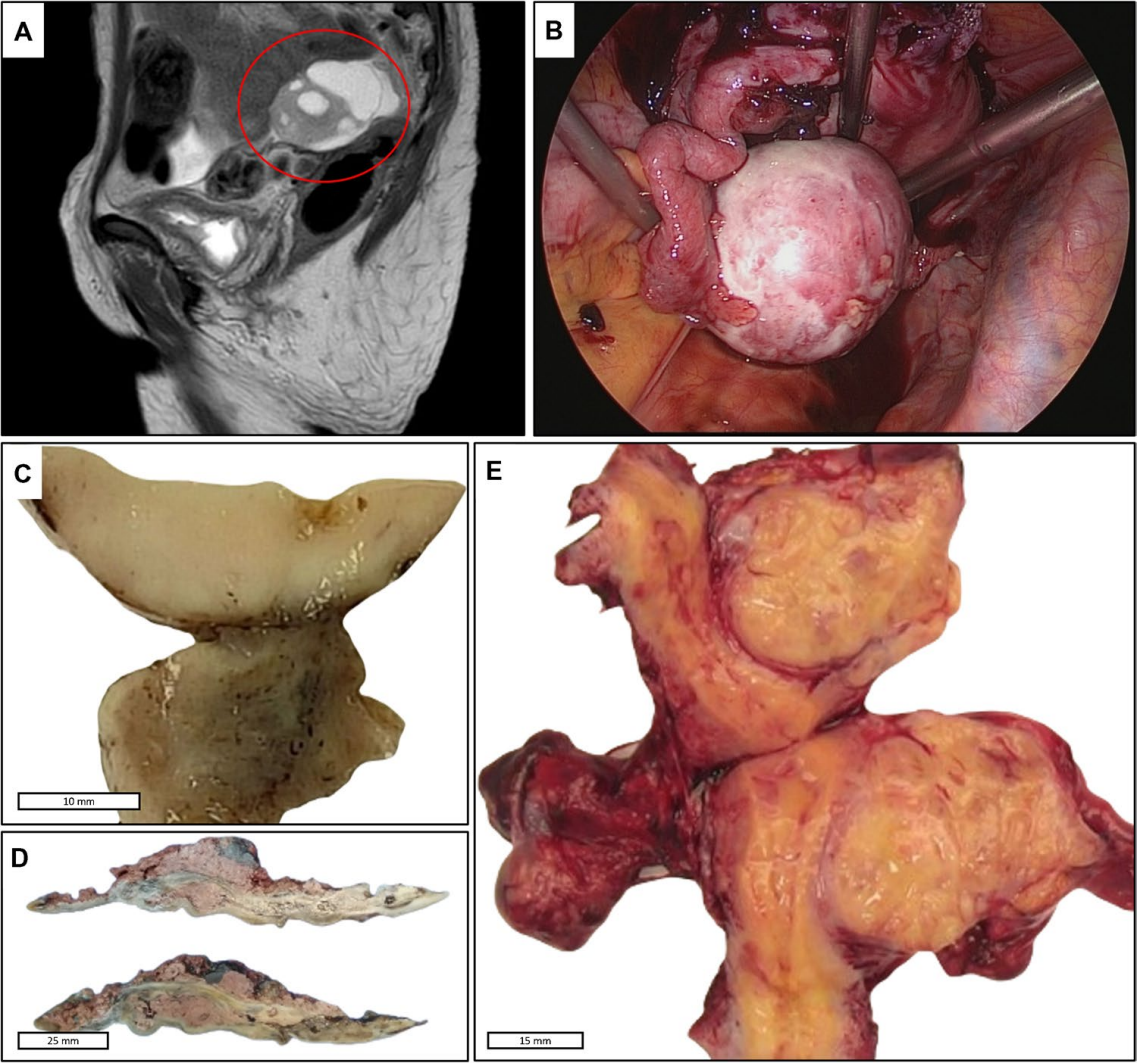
Radiological and gross findings in EWSR1/FUS-CREB-rearranged adnexal neoplasms. A (Case 1): sagittal T2-weighted pelvic MRI showed a 52-mm left adnexal mass (red circle) without peritoneal involvement. B (Case 3): coelioscopic view showing a solid periadnexal nodular mass. C (Case 1): gross examination revealed a solid nodule located on the surface of the ovary without infiltration of ovarian stroma. D (Case 2): gross examination demonstrated a hemorrhagic cystic lesion within the ovarian parenchyma reminiscent of an endometriotic cyst. E (Case 3): gross examination showed a 6 cm solid and yellow mass extending from the periadnexal tissue to the lateral wall of the myometrium
